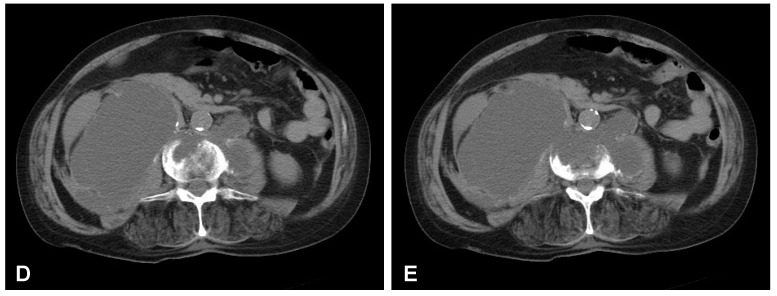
Preoperative sagittal (A–C) and axial (D,E) CT scans (non-contrast enhanced) of the lumbar spine demonstrating hypodense area at the L3/L4 intervertebral disc space communicating with both psoas major muscles forming massive well-defined fluid-like collection in the right retroperitoneum.
